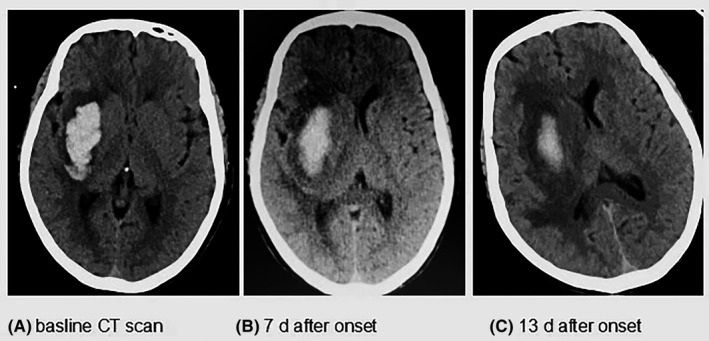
A, describes the sICH on the baseline CT scan within the 24 h after the symptom onset; B, shows the hematoma absorbed and the PHE increased at 7 d after the onset; C, shows us the area of PHE at 14 d is larger than the 7 d PHE but the HE is obviously decreased, named as DHE in our study
